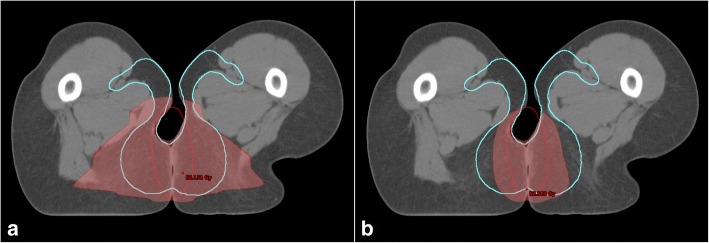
Axial CT slide 1.5 cm below the lower end of the anal canal in one patient. 30 Gy is represented in colorwash (red area). In this case, about 75% of ano-inguinal lymphatic drainage (cyan) is covered with 3D-RT (a) and just about 50% is covered using IMRT (b)
